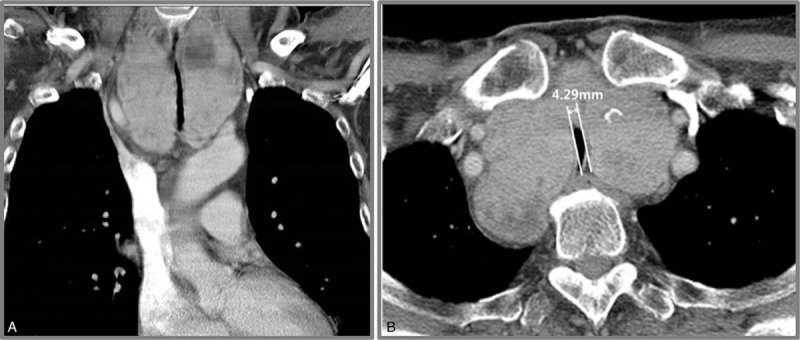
Preoperative CT findings for an elderly patient with a massive intrathoracic goiter causing severe tracheal obstruction with positional symptoms. CT shows a large multinodular goiter extending to the thoracic cavity, with diffuse segmental tracheal narrowing for 6 cm (A). The diameter at the narrowest portion of the trachea is 26 × 4.29 mm (B). CT = computed tomography.
